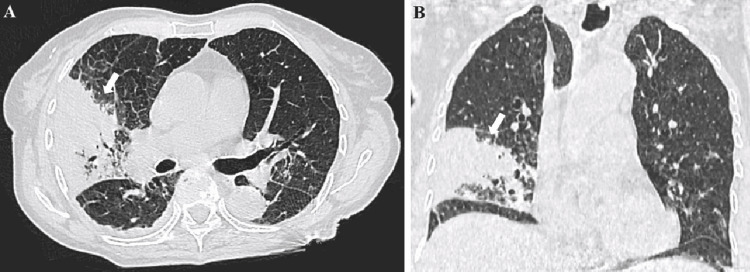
Unenhanced chest CT axial section (A) and sagittal section (B): consolidation of the middle lower lobe with air bronchogram and presence of several pseudonodular condensation areas
